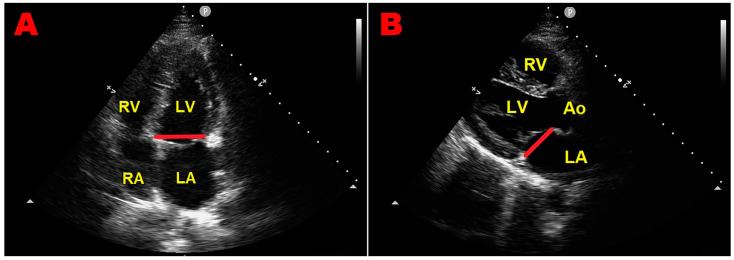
An example of superior anterior mitral leaflet displacement in the apical four-chamber view (panel (A)), absent in the parasternal long-axis view (panel (B)), accepted as the diagnostic standard of mitral valve prolapse before the year 1999. The red line indicates the mitral annular plane in each of the two orthogonal views. Ao, aorta; LA, left atrium; LV, left ventricle; RA, right atrium; RV, right ventricle.
